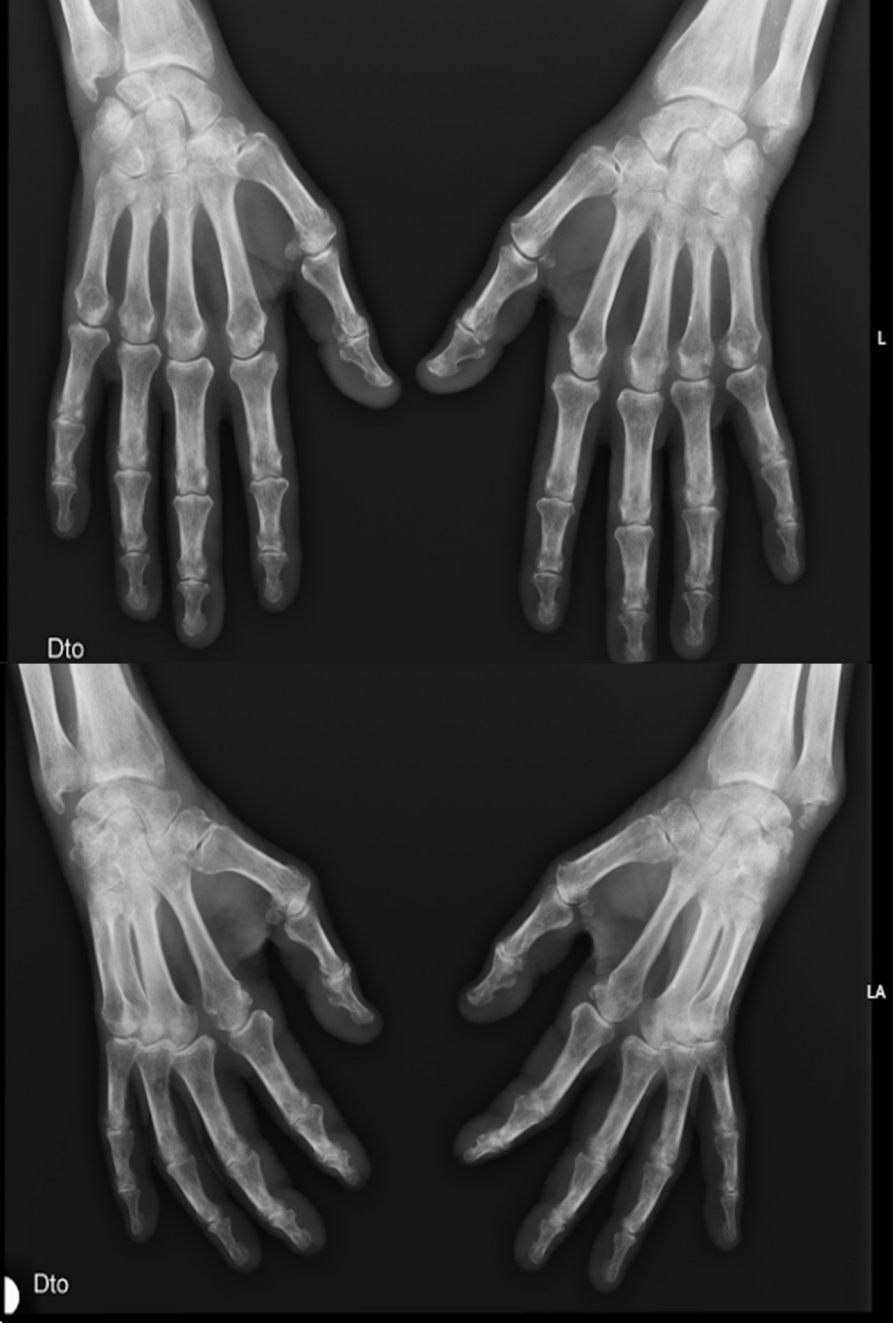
Radiographs of the hands (posteroanterior, superior; oblique, inferior)*.
